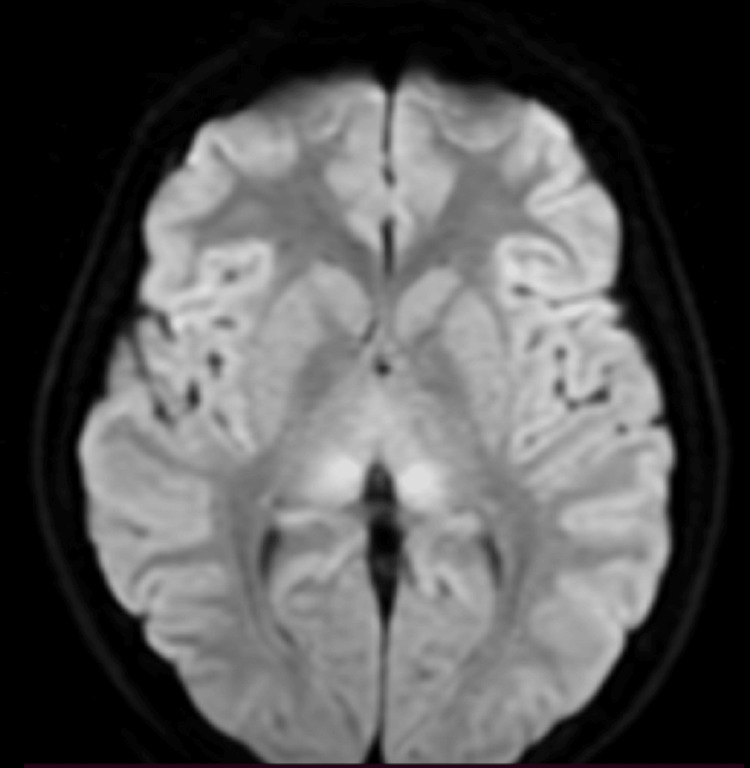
MRI brain revealed areas of hyperintense signal with symmetric involvement of the posterior thalami.
